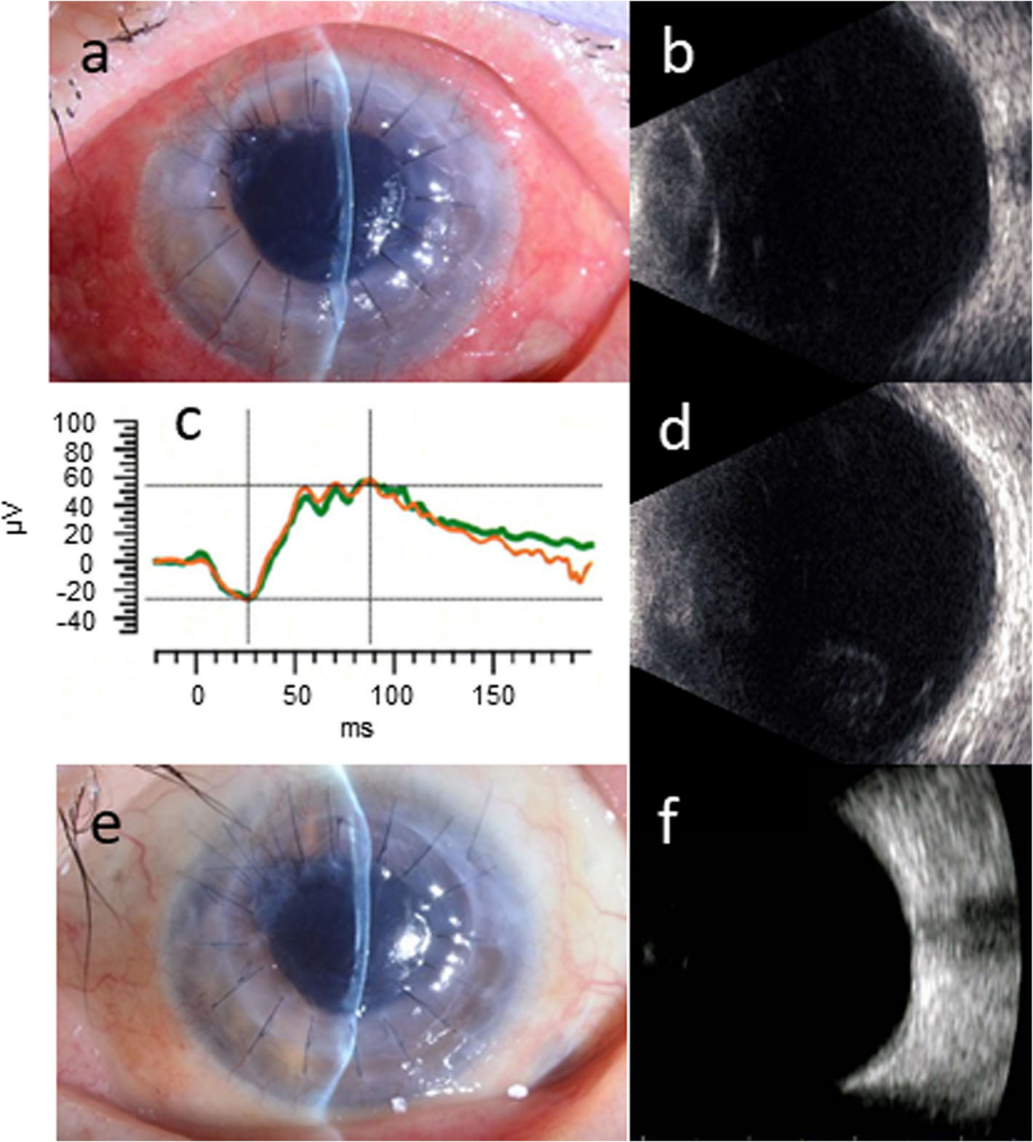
a Hypopyon and corneal infiltration were both absent 3 weeks after the surgery. (b and c) Vitreous opacity was largely absent on B-scan ultrasonography, and the full-field ERG waveform acquired at the same time had no amplitude reduction or latency delay. d Although mild vitreous opacity was confirmed on ultrasonography 4 days after tocilizumab infusion, it spontaneously improved. e and f There were no signs of infection relapse in the slit-lamp examination, and no vitreous opacity on B-scan ultrasonography 4 months after the surgery
